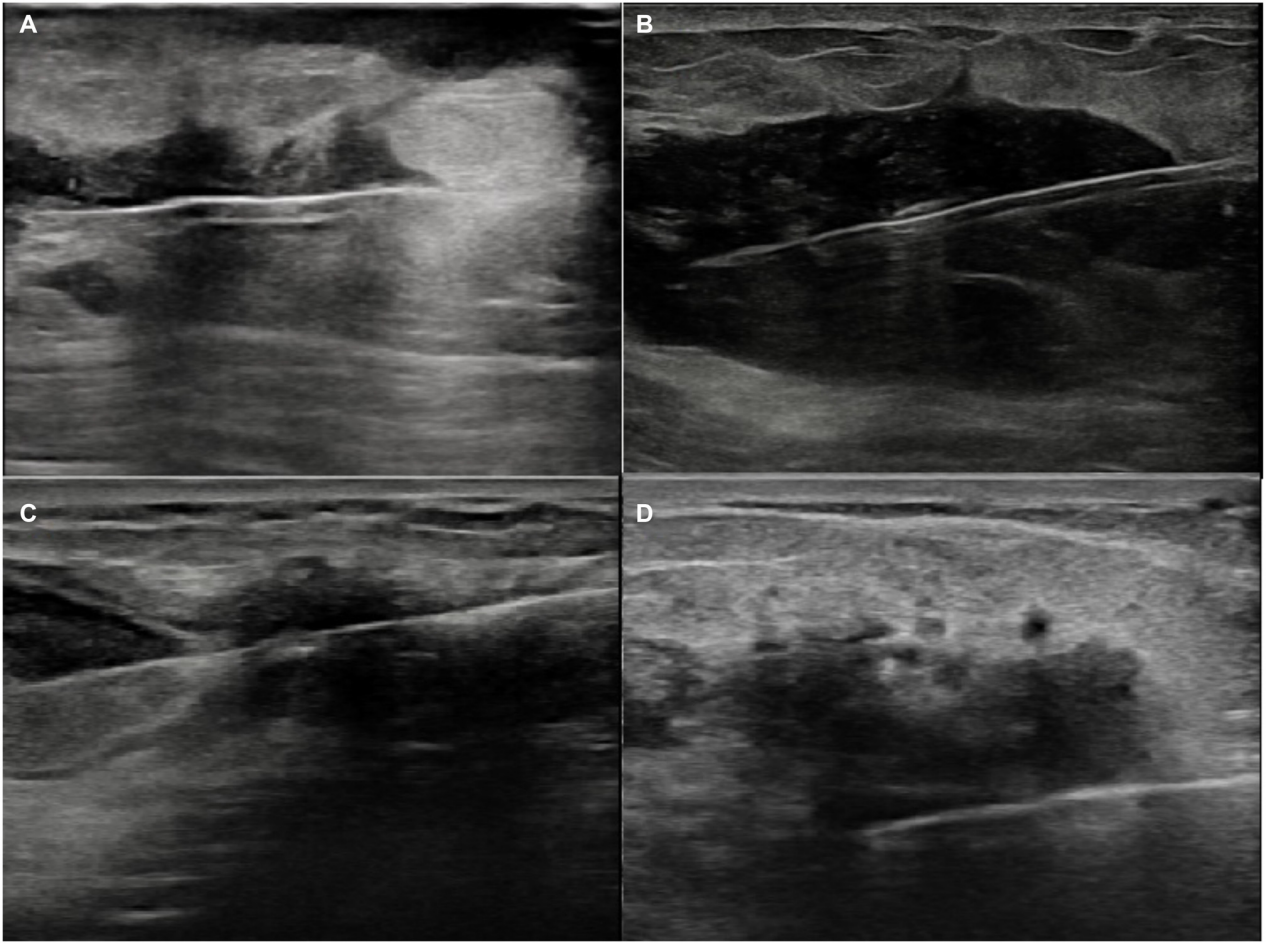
USG-guided tru-cut biopsy images. (A–D) Depicting USG-guided tru-cut biopsies of lesions within the breast parenchyma from four different patients diagnosed with idiopathic granulomatous mastitis. USG: Ultrasonography.
